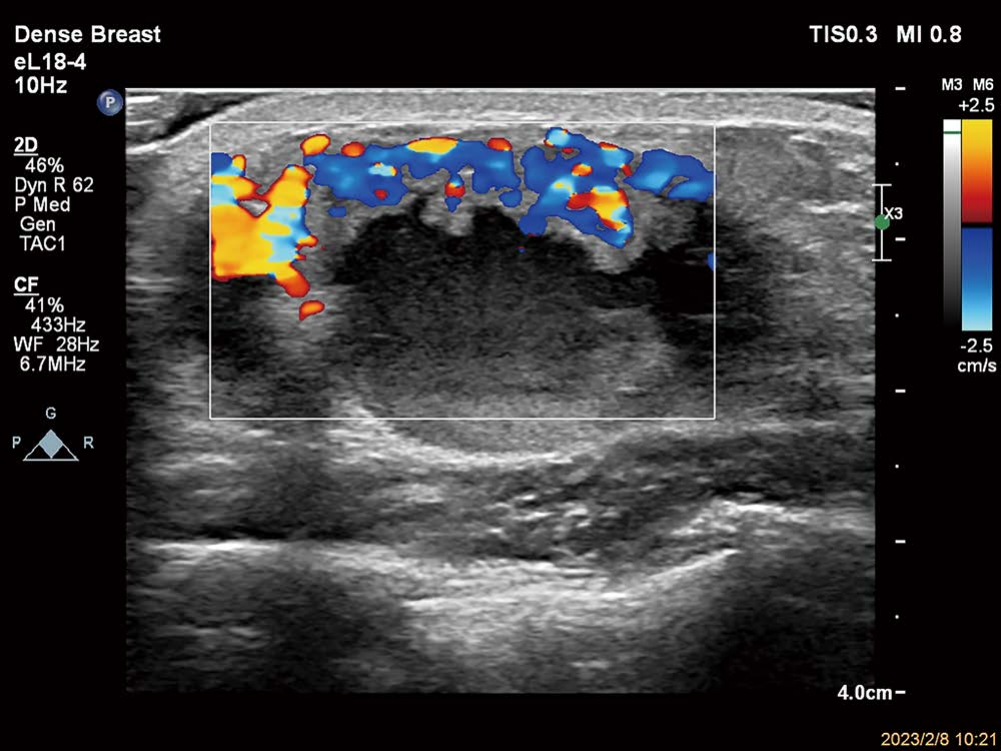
Color Doppler flow imaging (CDFI) showing abundant blood flow signals in the solid echogenic portion. CDFI = color doppler flow imaging.
